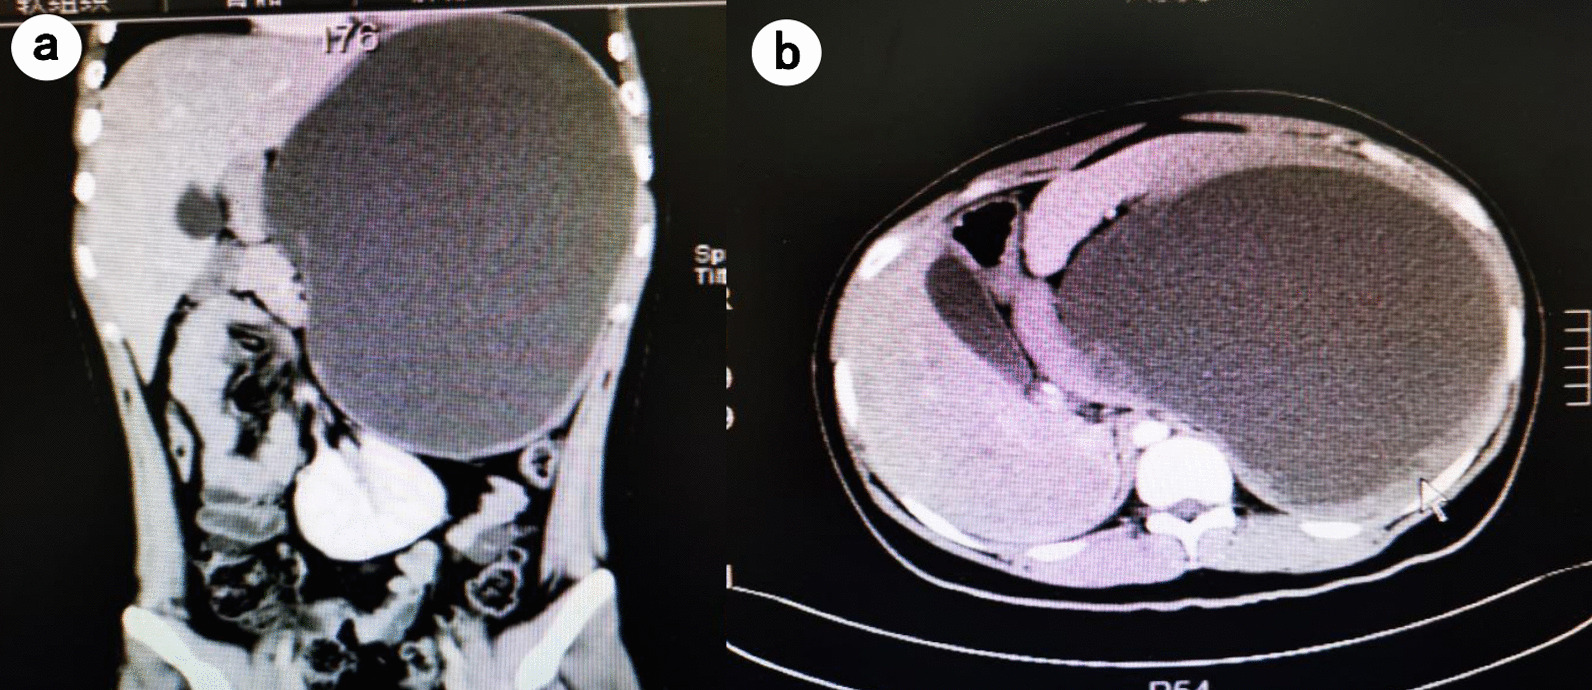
Abdominal CT taken at the local hospital 6 days prior to admission showed a 21 × 16 cm giant abscess in spleen
